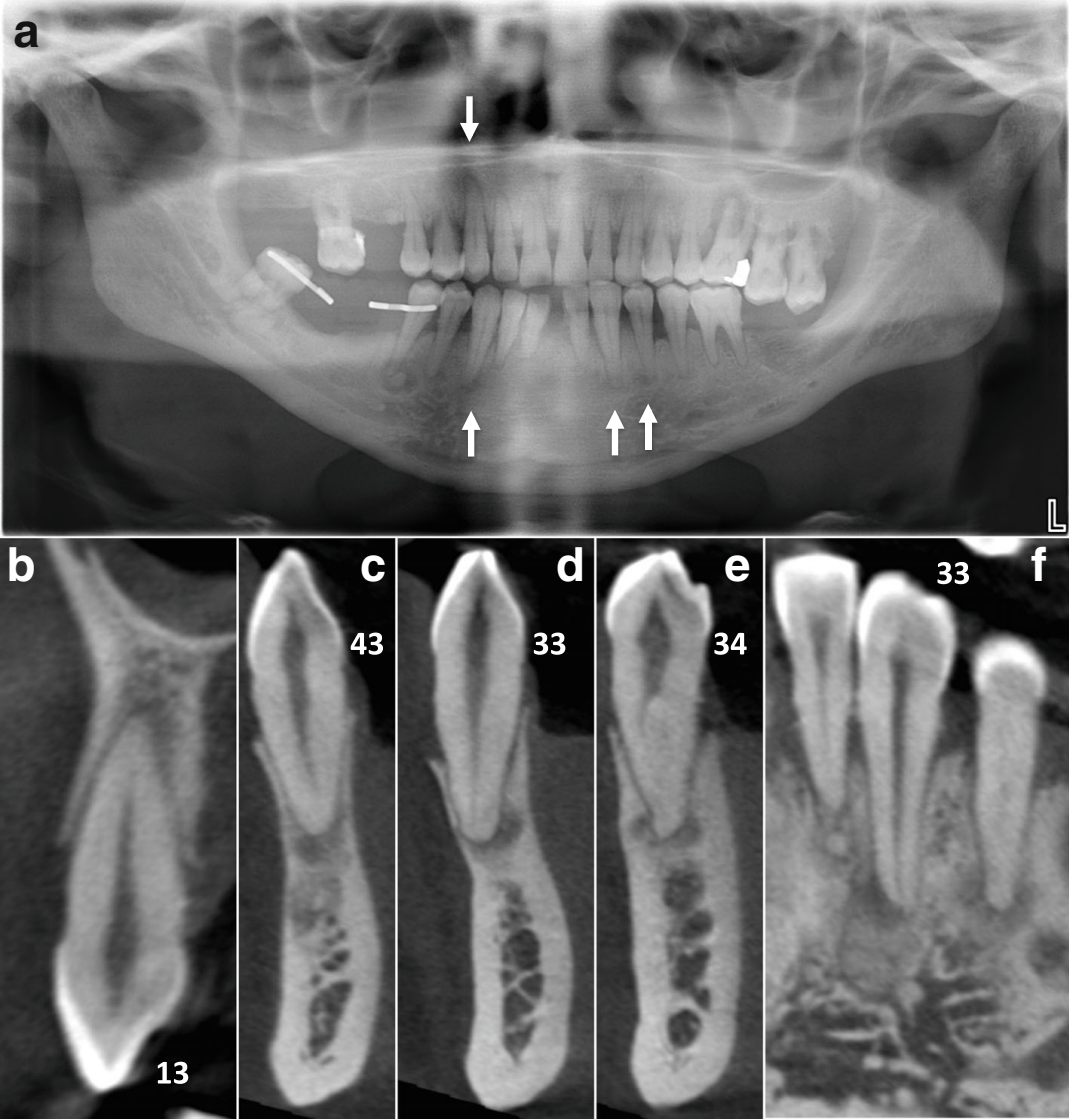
Stage 1 FOD with periapical involvement. Multiple periapical radiolucent lesions (white arrows) on vital teeth mimicking periapical endodontic lesions are observed on panoramic radiography (a) and detailed by CBCT cross sections focused on 13 (b), 43 (c), 33 (d), and 34 (e and f)
